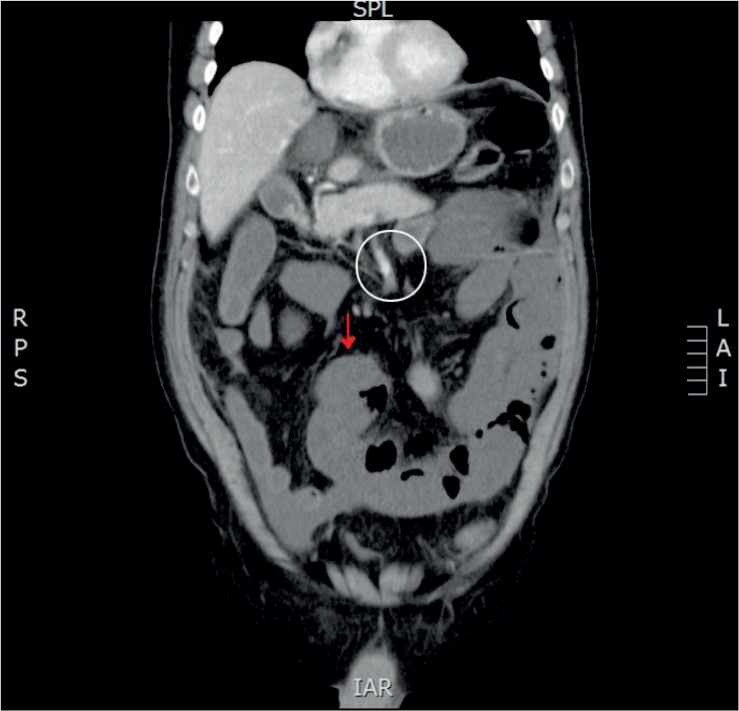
Image of a CT slice which confirmed superior mesenteric artery thrombosis (white circle) and resultant ischemia without contrast enhancement of the bowel wall (red arrow). For comparison there are some bowel loops with contrast enhancement of the bowel wall visible in the upper left part of the image.
